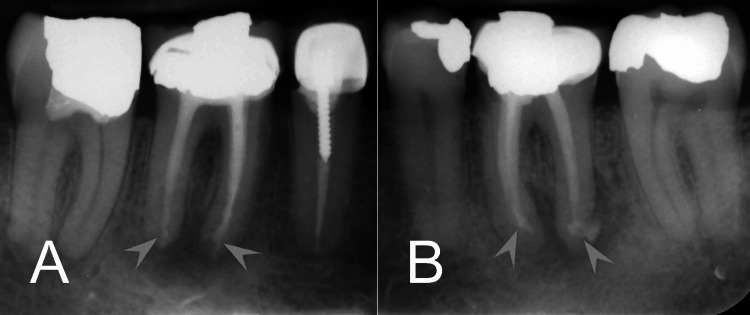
Immediate postoperative radiographs after bio-obturation with CEM cement. (A) Tooth #46 demonstrating well-adapted root canal filling with no visible gaps or complications. The IRRs were completely filled/sealed with bio-obturation (arrowheads) (B) Tooth #36 showing similarly successful bio-obturation with proper sealing of the root canal system and resorptive defects (arrowheads). CEM: calcium-enriched mixture; IRR: internal root resorption
